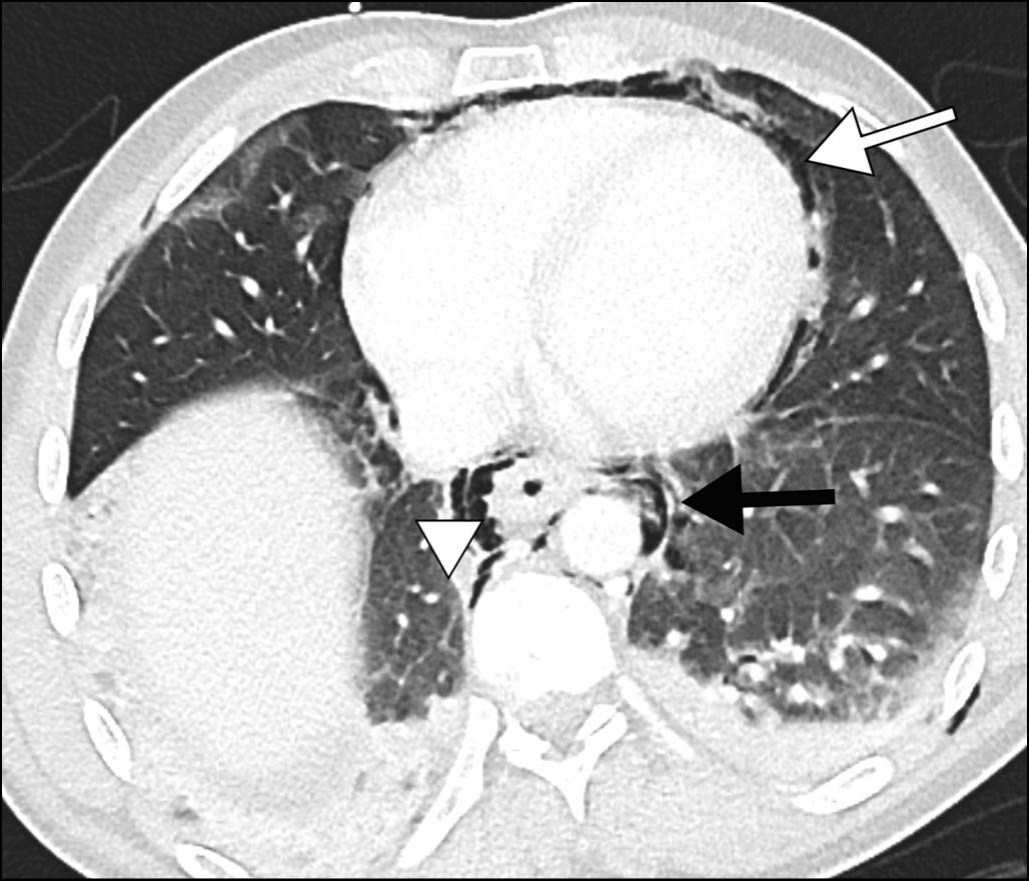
Contrast-enhanced computed tomography showing an extensive pneumomediastinum with pneumopericardium and subcutaneous emphysema of the anterior thoracic wall and neck.
